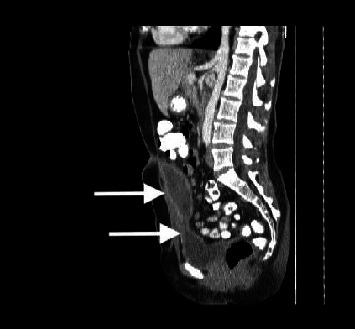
Contrast–enhanced computed tomography (sagittal plane) showing the cystic lesion (arrow-above) arising from the bladder dome (arrow–below) and extending to the umbilical region, leading to a suspected diagnosis of an infected vesicourachal diverticulum.
